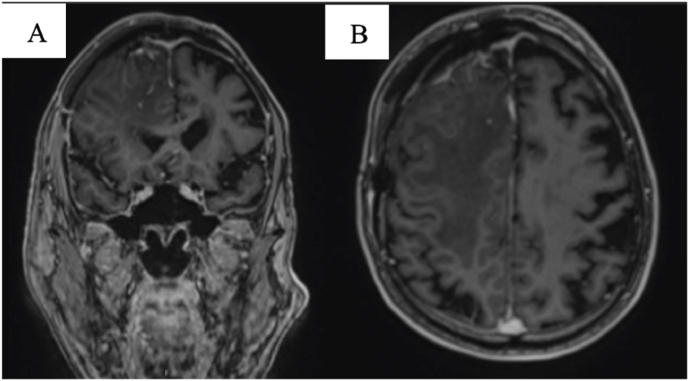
Contrast-enhanced T1-weighted images after surgery. A: MRI in coronal plane. B: MRI in axial plane.
